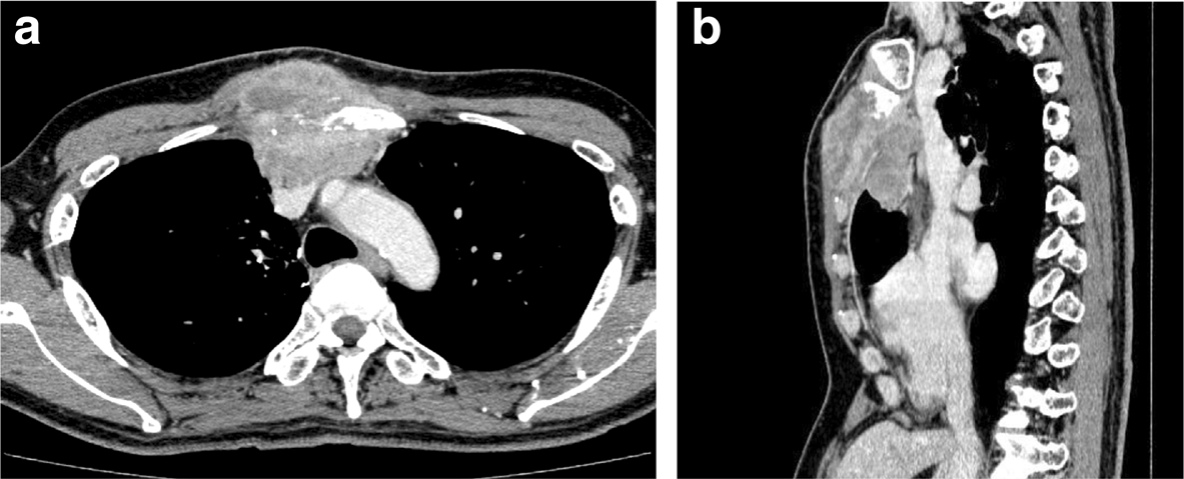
The computed tomographic scan showed an anterior mediastinal tumor penetrating into the sternum. (a) Horizontal section; (b) sagittal section.
